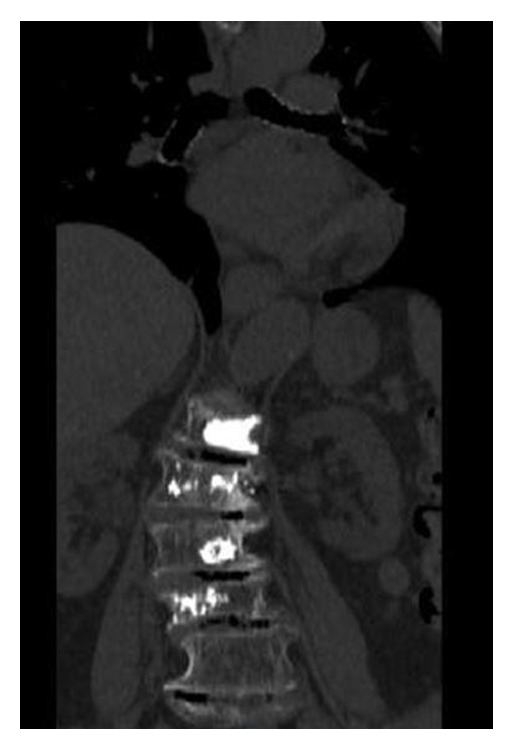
Computed Tomography scan, coronal reconstruction (different patient) after vertebroplasty. There are no cement leakages and PMMA is distributed in the inner side of the scoliotic arch. Notice the multilevel intradiscal vacuum phenomenon due to extensive spinal degeneration.
